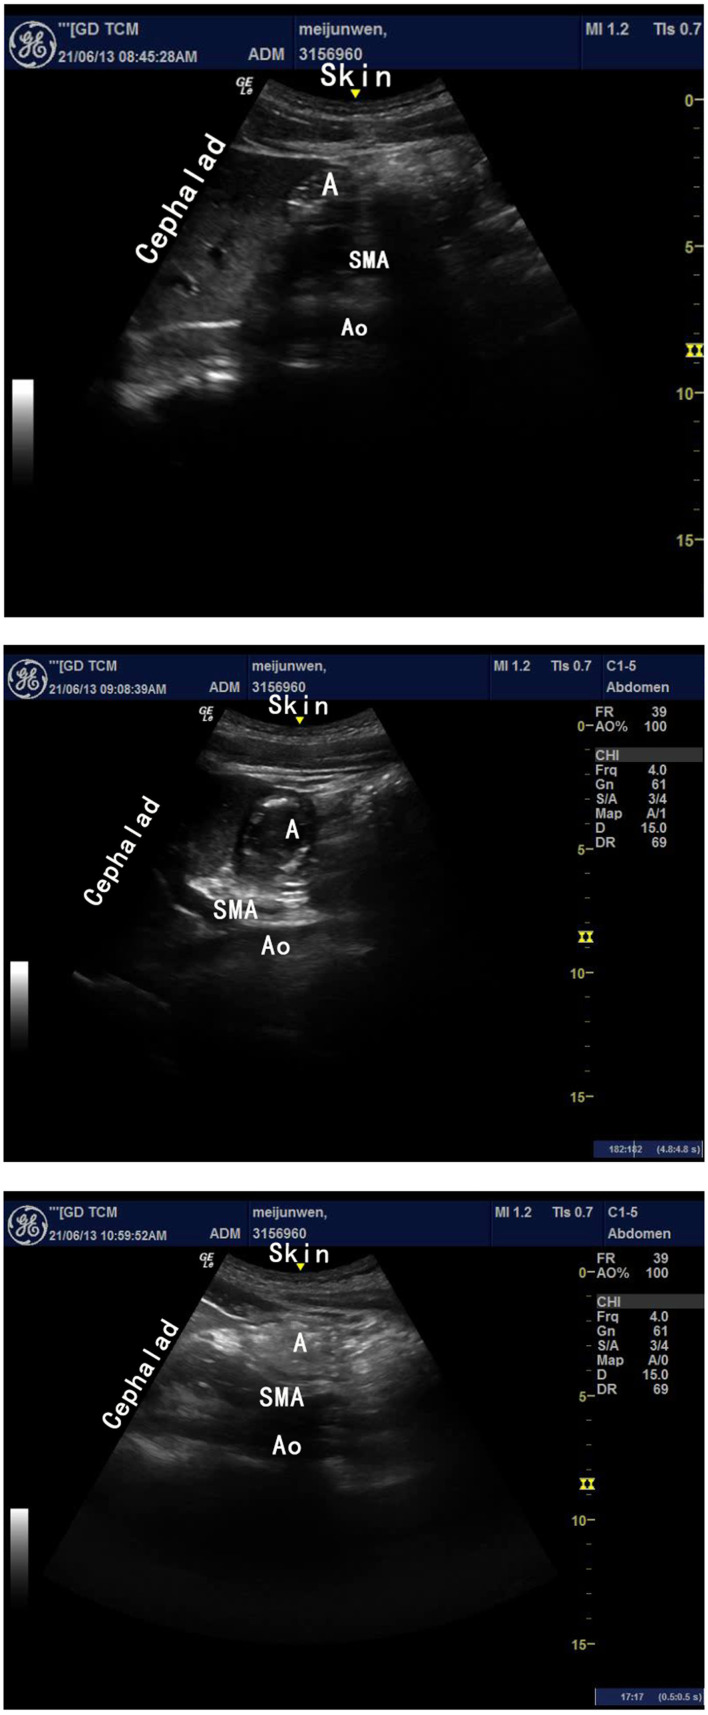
(A–C) Represent the ultrasound images of different gastric contents. (A) Empty, (B) Clear fluid, (C) Solid; SMA, superior mesenteric artery; Ao, aorta abdominalis; A, antrum of stomach.
